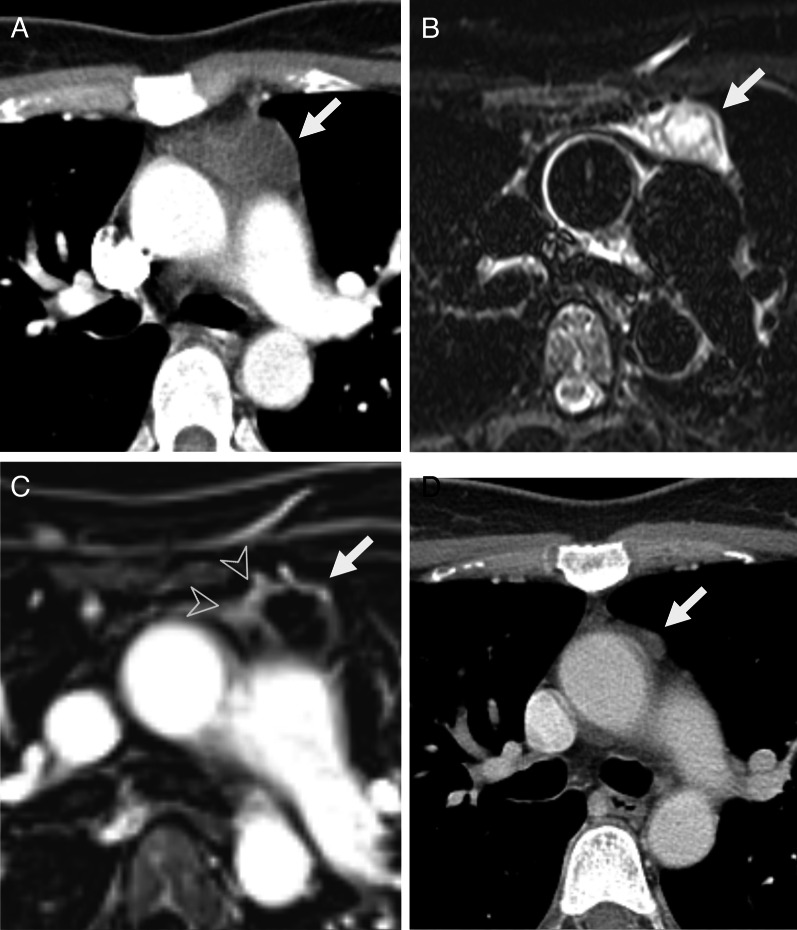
Follow-up outcome of 46-year-old female with anterior mediastinal cystic lesion who underwent chest MRI. Initial contrast-enhanced chest CT shows a 46 mm anterior mediastinal mass with heterogeneous attenuation (arrow, a). Patient underwent chest MRI after 2 weeks. On chest MRI, the lesion shows mostly high signal intensity with linear dark signal intensity septa on axial T2-weighted images (arrow, b), suggesting multilocular cystic lesion. On subtraction images of axial post- and pre-contrast T1-weighted images, the lesion shows enhancing wall (arrow, c) with irregular and eccentric wall thickening (arrowhead, c). The lesion can be classified as an indeterminate lesion (complex cyst) based on chest MRI findings and the differential diagnosis of MRI reading included complicated thymic cyst and cystic thymoma. Patient underwent 3-month follow-up after the MRI rather than immediate surgery. On 3-month follow-up CT, the lesion shows a marked decrease in size of anterior mediastinal lesion (arrow, d), which suggests the benign nature of lesion, complicated thymic cyst
